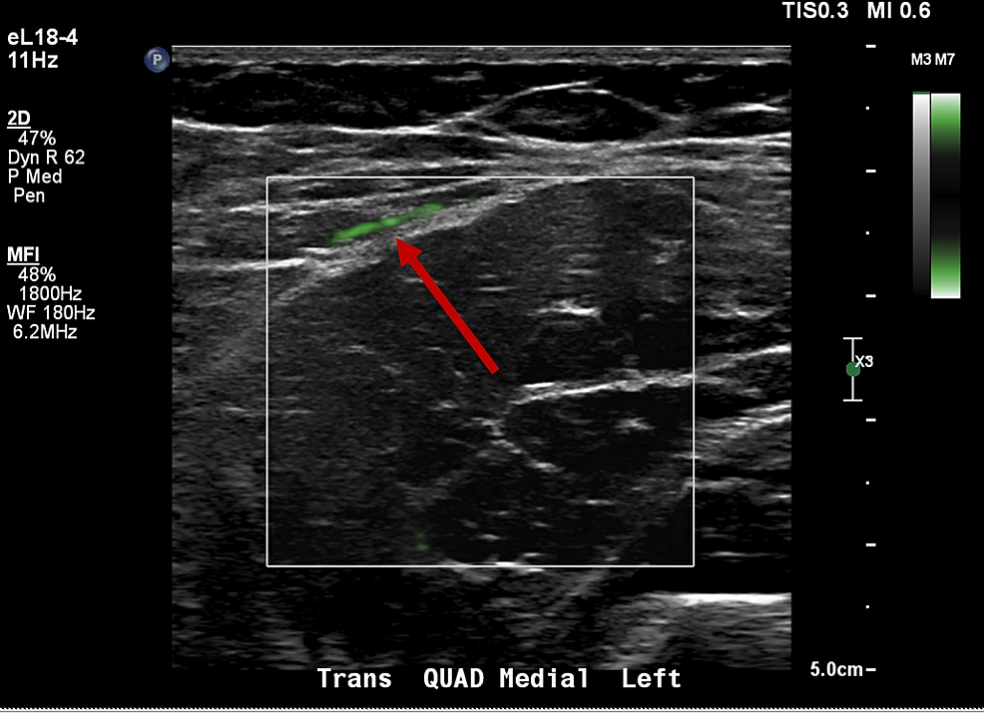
Power Doppler ultrasound: transaxial view of the quadriceps muscle (square) showing typical Doppler pattern (green) in cases of active myositis flare
